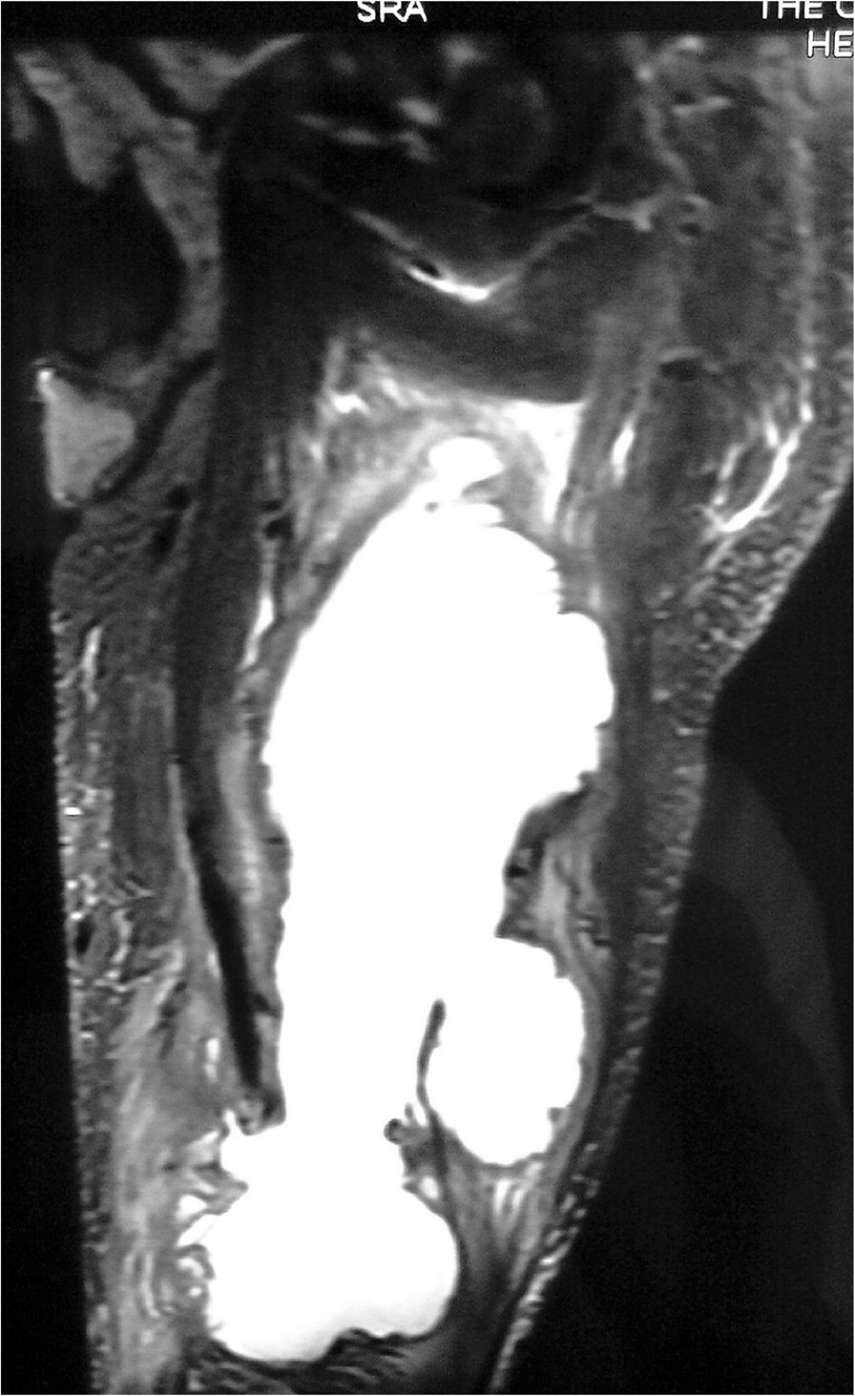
T2-weighted magnetic resonance image of the left thigh shows pyomyositis. T2-weighted magnetic resonance image of the left thigh taken in the sagittal plane reveals a multi-loculated mass in the medial aspect that demonstrates high signal intensity.
